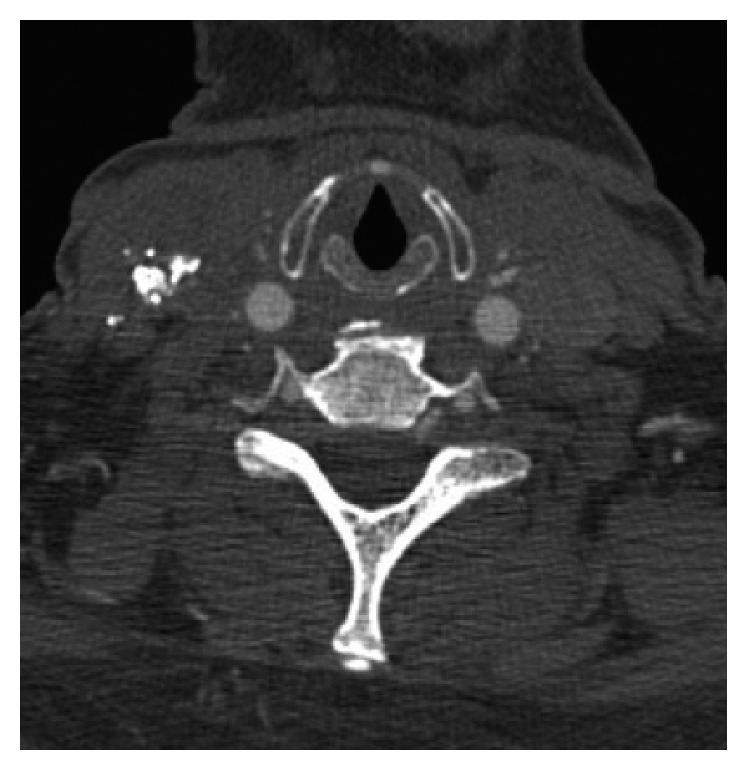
CT angiography neck: right neck mass on 1 month follow-up status after right pseudoaneurysm embolized with glue. These can be compared to the preintervention CT in Figure 1 showing that the neck mass has improved.
